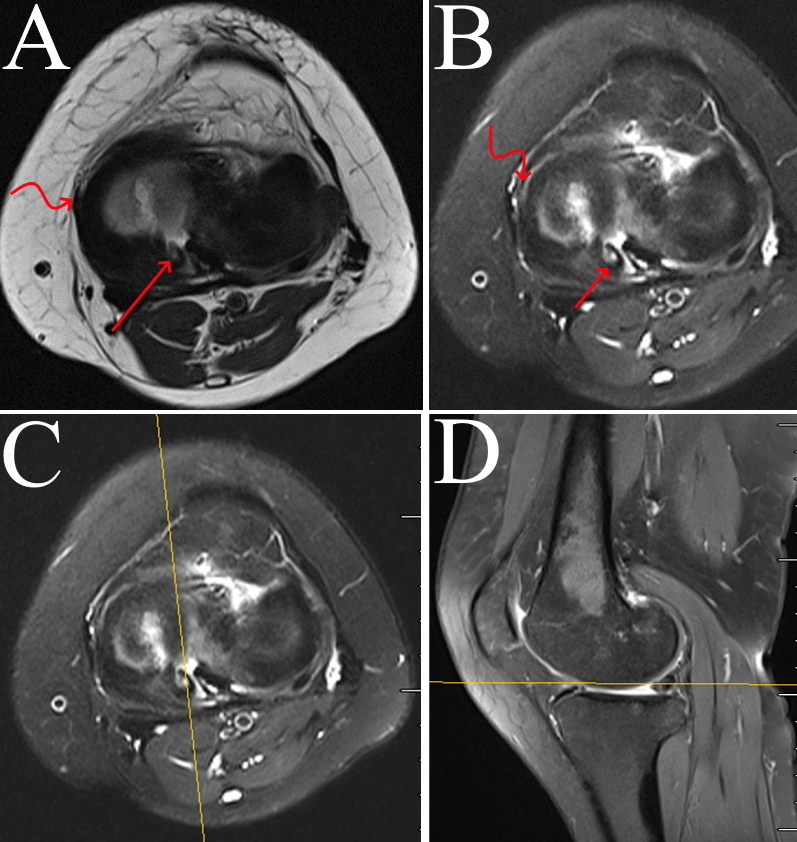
(A, B) Axial MRI scan showing medial meniscus (curved arrow) and meniscal ossicle (straight arrow) (C, D) location of the meniscal ossicle on axial and sagittal MRI scans.
